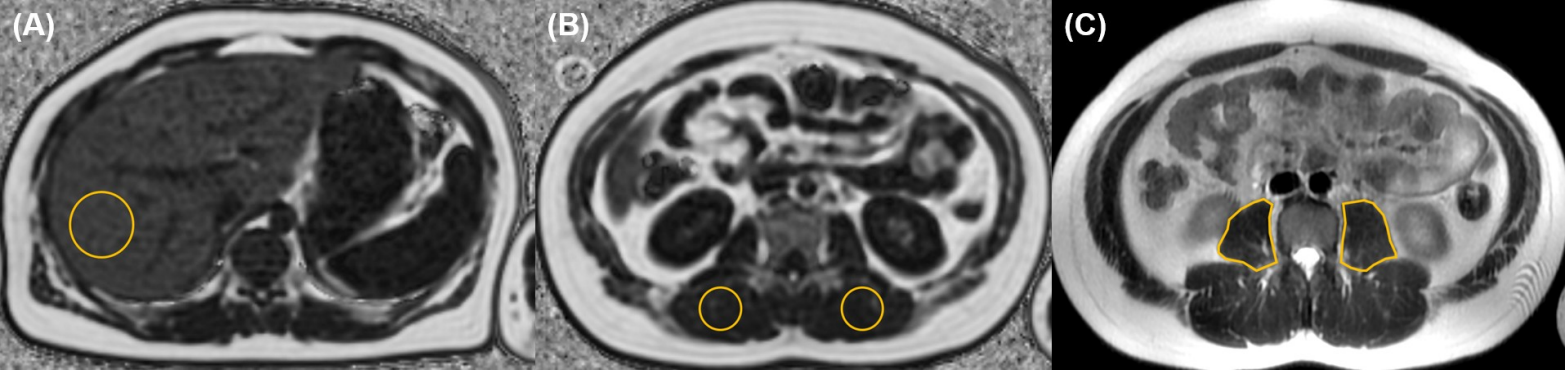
A 14-year-old boy with obesity (body mass index, 28.9 kg/m^2^) and fatty liver. From the liver MRI, (A) fat fraction of the liver (18%) was obtained from oval or circular region-of-interest (ROI, yellow circle) in the right lobe on the fat map. (B) The ROIs (yellow circles) for paraspinal muscle fat fraction were drawn bilaterally at the level of mid L2 and the mean value was 3.2% in this case. (C) In order to measure the psoas muscle area (PMA), free hand ROIs (yellow lines) were drawn at the level of mid L3 on T2-weighted axial images. The PMA was 1197.7 mm^2^ and the z-score of PMA was -2.56 in this patient.
